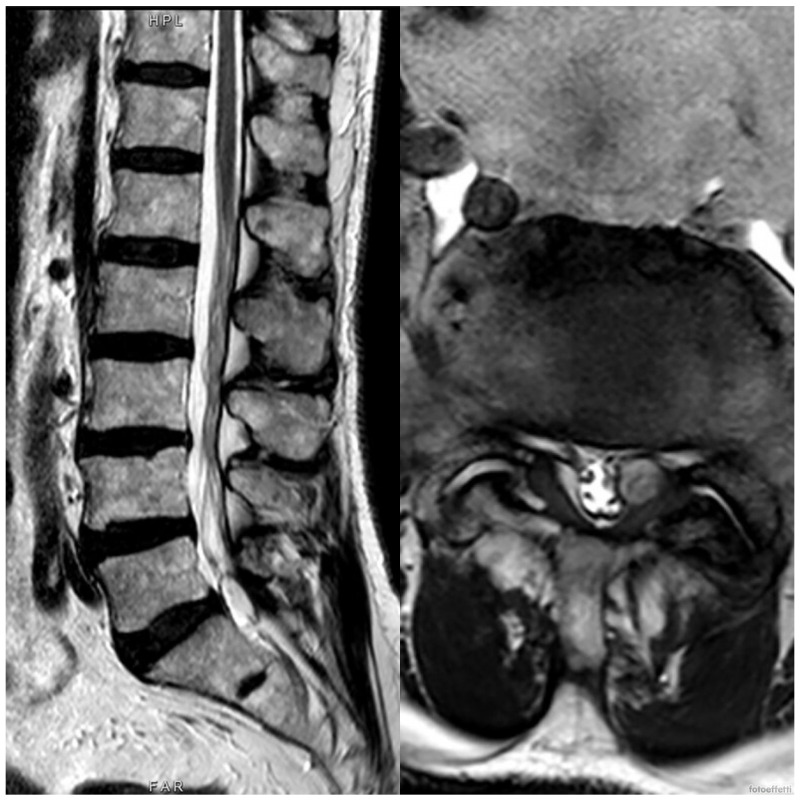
Preoperative MRI of the lumbar spine of the first case, a 66-year-old man, where there is a synovial cyst at L5-S1 level.
